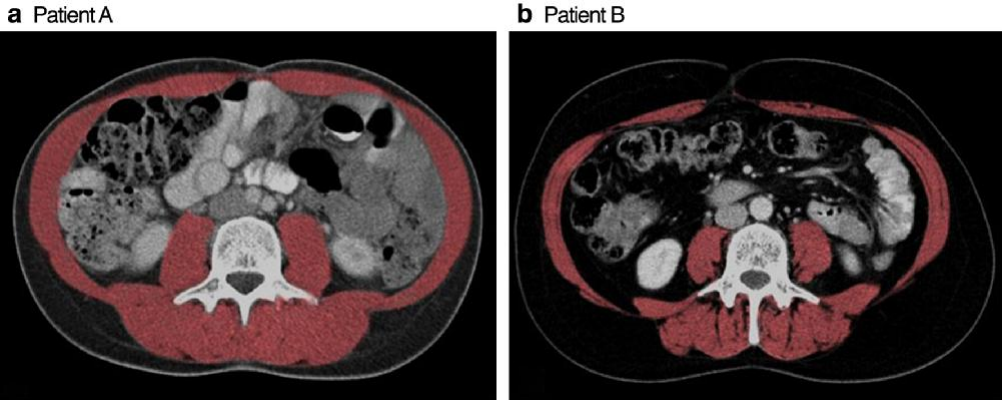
Computed tomography assessment of body composition Abdominal computed tomography for two patients with breast cancer. a Patient A had a BMI of 22.8 kg/m^2^; b Patient B had a BMI of 23.7 kg/m^2^. Despite similar BMIs, Patient A has normal lean tissue and visceral fat areas, whereas Patient B has both visceral obesity and sarcopenia. BMI, body mass index.
